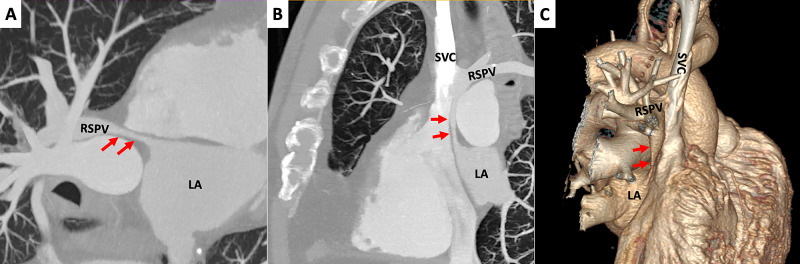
Cardiac computed tomography: surgically created baffle (A,B) Maximum intensity projection (MIP) images in oblique plane and coronal plane, respectively. It is possible to see the baffle (arrows) connecting the right superior pulmonary vein (RSPV) to the roof of left atrium (LA). Furthermore, in (B), we can also see again RSPV draining into superior vena cava (SVC). (C) Volume rendering images. In this 3D image it is possible to evaluate the course of the baffle, from inferior aspect of RSPV to the roof of LA.
